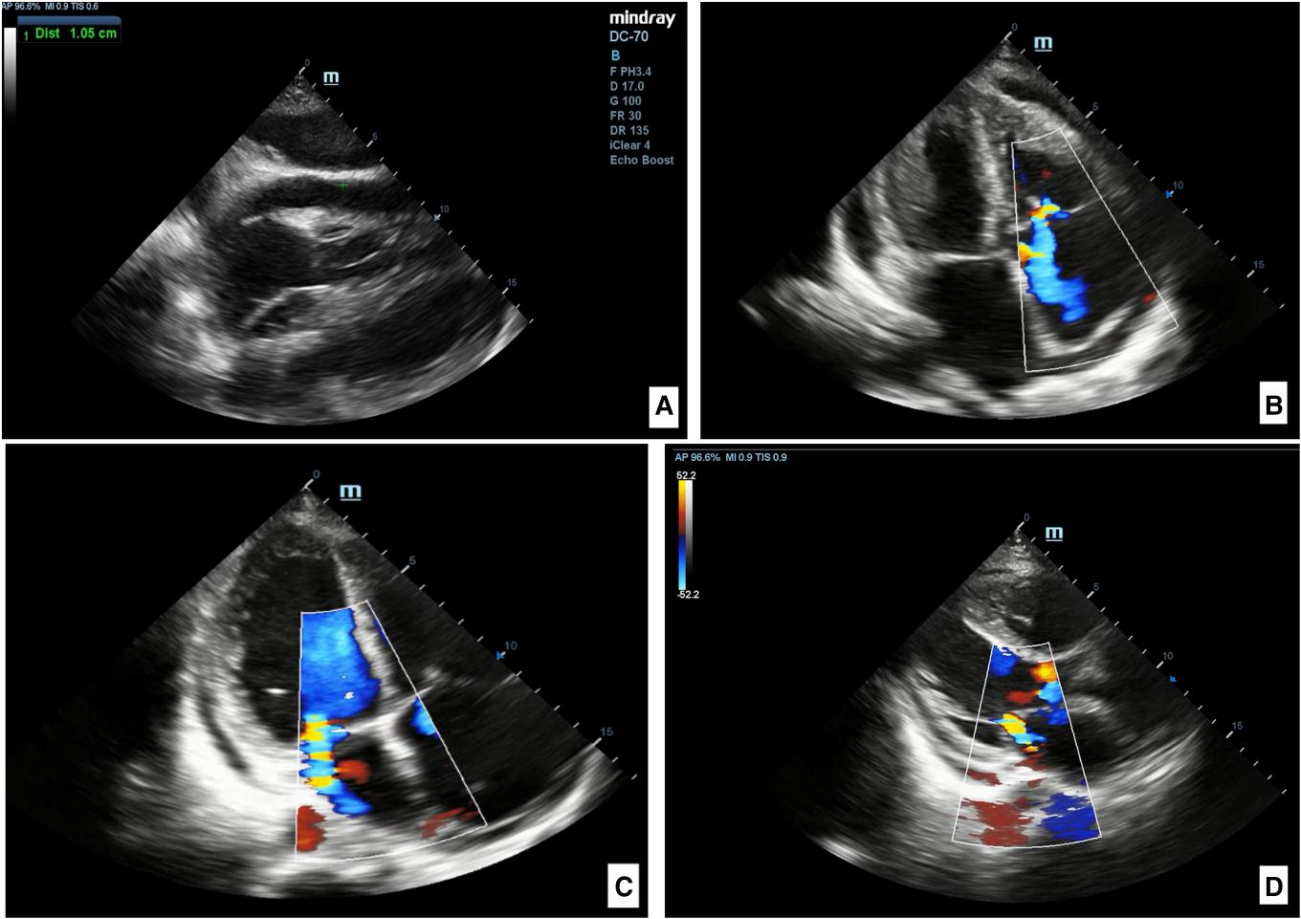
Echocardiography: (*A*) pericardial effusion, (*B*) moderate-to-severe tricuspid regurgitation, and (*C*, *D*) moderate mitral regurgitation.
